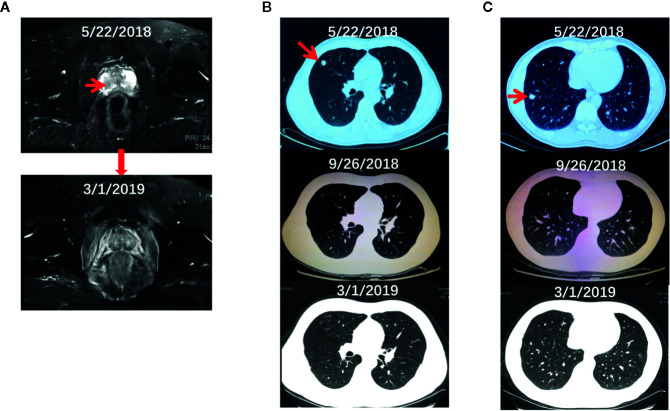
The radiographs of the proband before and during the treatment. (A–C) The pelvic MRI (A) and chest CT (B, C) scan of the proband before and after the systematic treatment.
